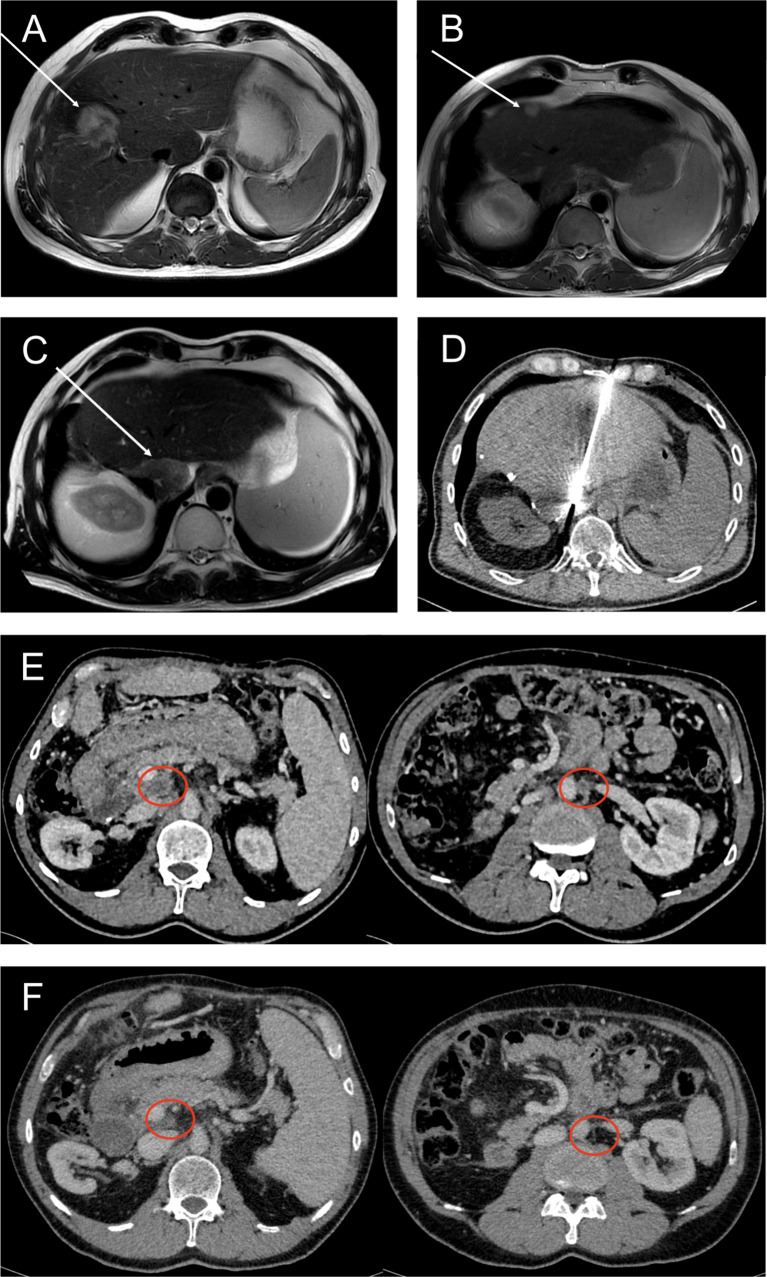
Representative CT and MRI scans showing different disease stages and local ablative therapy during the disease course. (A) MRI (T2) of July 2017. White arrow points toward intrahepatic cholangiocarcinoma in segment V/VIII at first presentation. (B) MRI (T2) of October 2018 revealing a singular hepatic metastasis (white arrow). (C) Second intrahepatic recurrence of CCA (white arrow) diagnosed by MRI (T2) in February 2020. (D) CT-guided microwave ablation of this second hepatic metastasis in February 2020. (E) CT (portal venous phase) showing central necrotic lymph node metastases (red circles) in October 2020, before initiating olaparib/pembrolizumab combination therapy. (F) In July 2021, CT scan (portal venous phase) shows partial and complete remission of lymph node metastases after about 9 months of treatment with olaparib and pembrolizumab.
